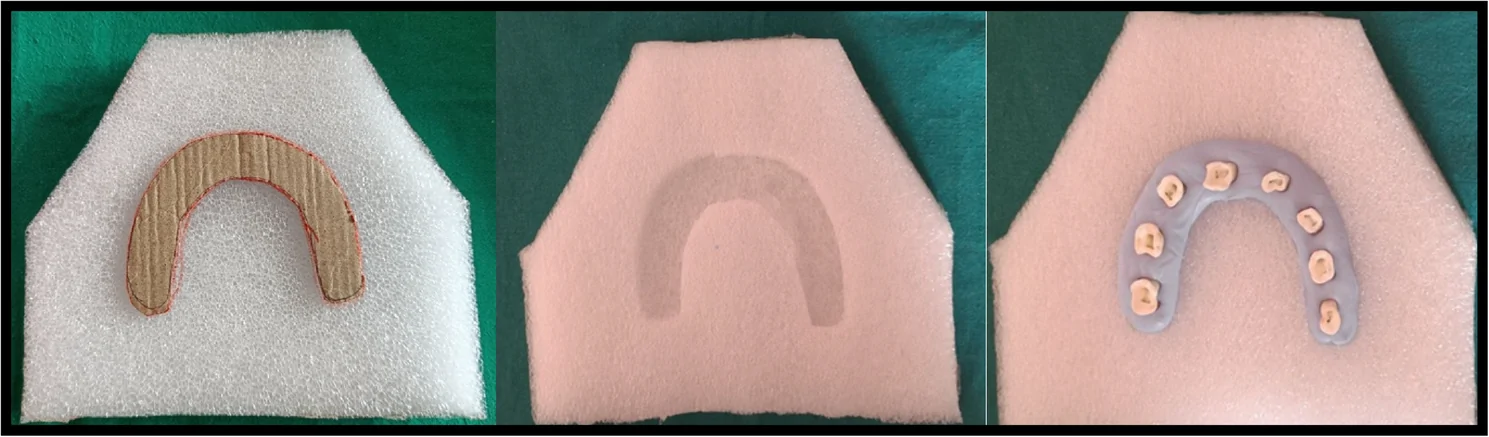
Indexing of foam sheet to accommodate impression material and sample teeth mounted for CBCT scanning
